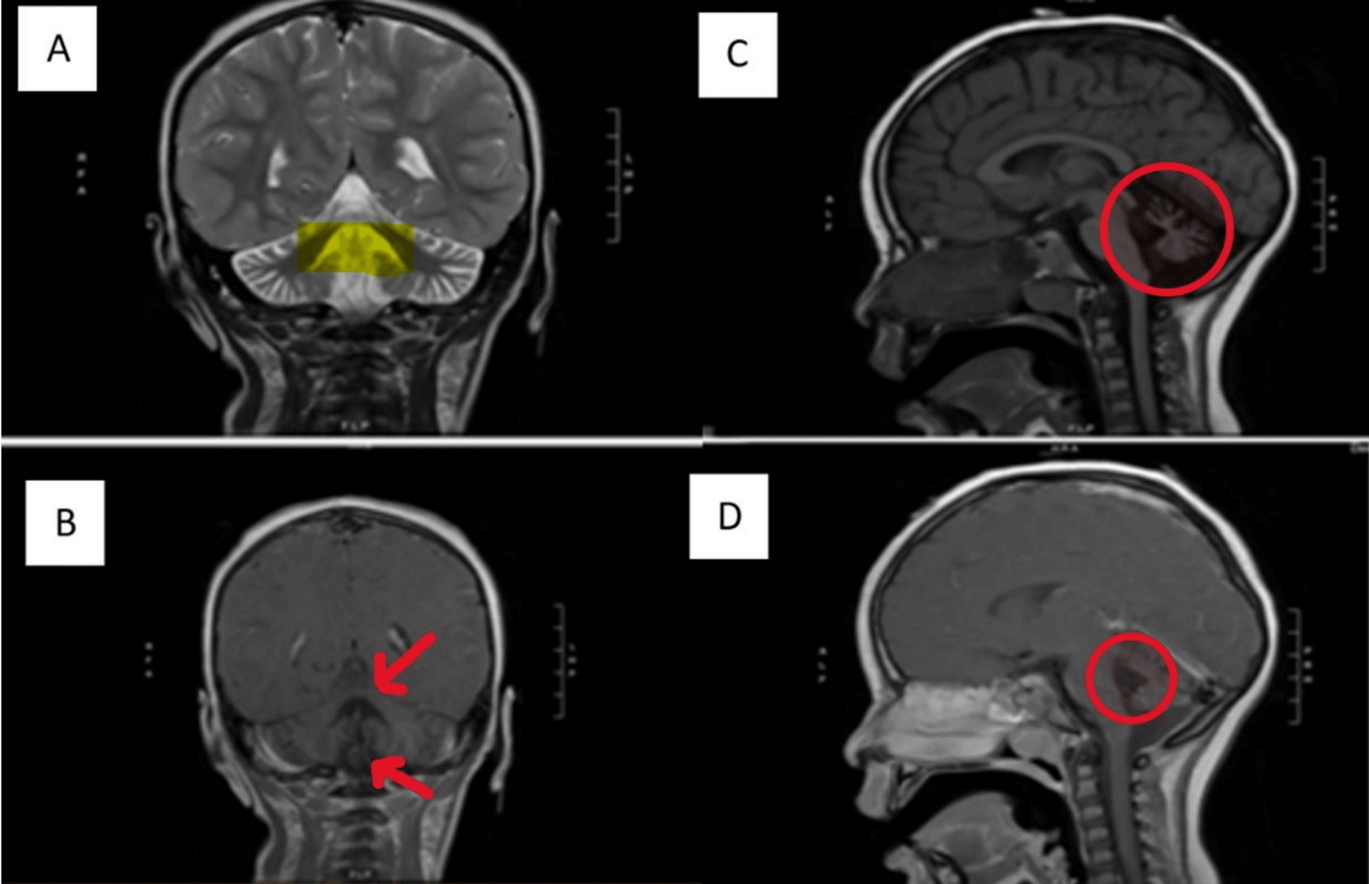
Brain MRI findings Brain MRI shows cerebellar atrophy, most pronounced in the vermis, with ex-vacuo dilation of the fourth ventricle. Figure A presents the coronal T2 view. The yellow highlighted area shows an enlarged fourth ventricle. Figure B presents coronal T1 post-contrast view. The red arrows depict atrophy of the superior and inferior vermis. Figure C presents sagittal T1 FLAIR view. As shown in this red circle, the cerebellum does fully occupy its allotted space. Figure D presents sagittal T1 post-contrast view. The fourth ventricle is dilated, as evidenced by the increased hypointense area within the red circle.
